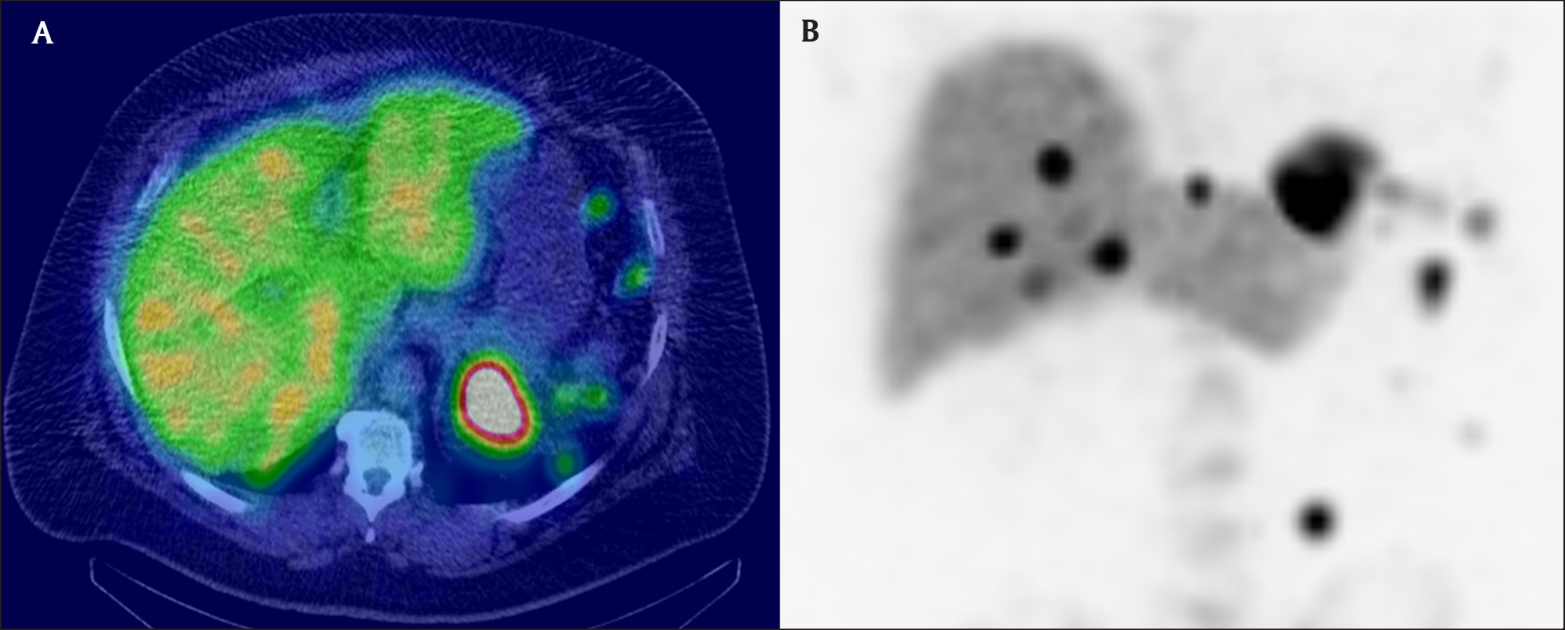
Fusion Tc99m-DRBC + CT axial slice (A) and coronal maximal intensity projection reconstruction of the scintigraphy (B) demonstrating uptake of these multiples nodular lesions.
